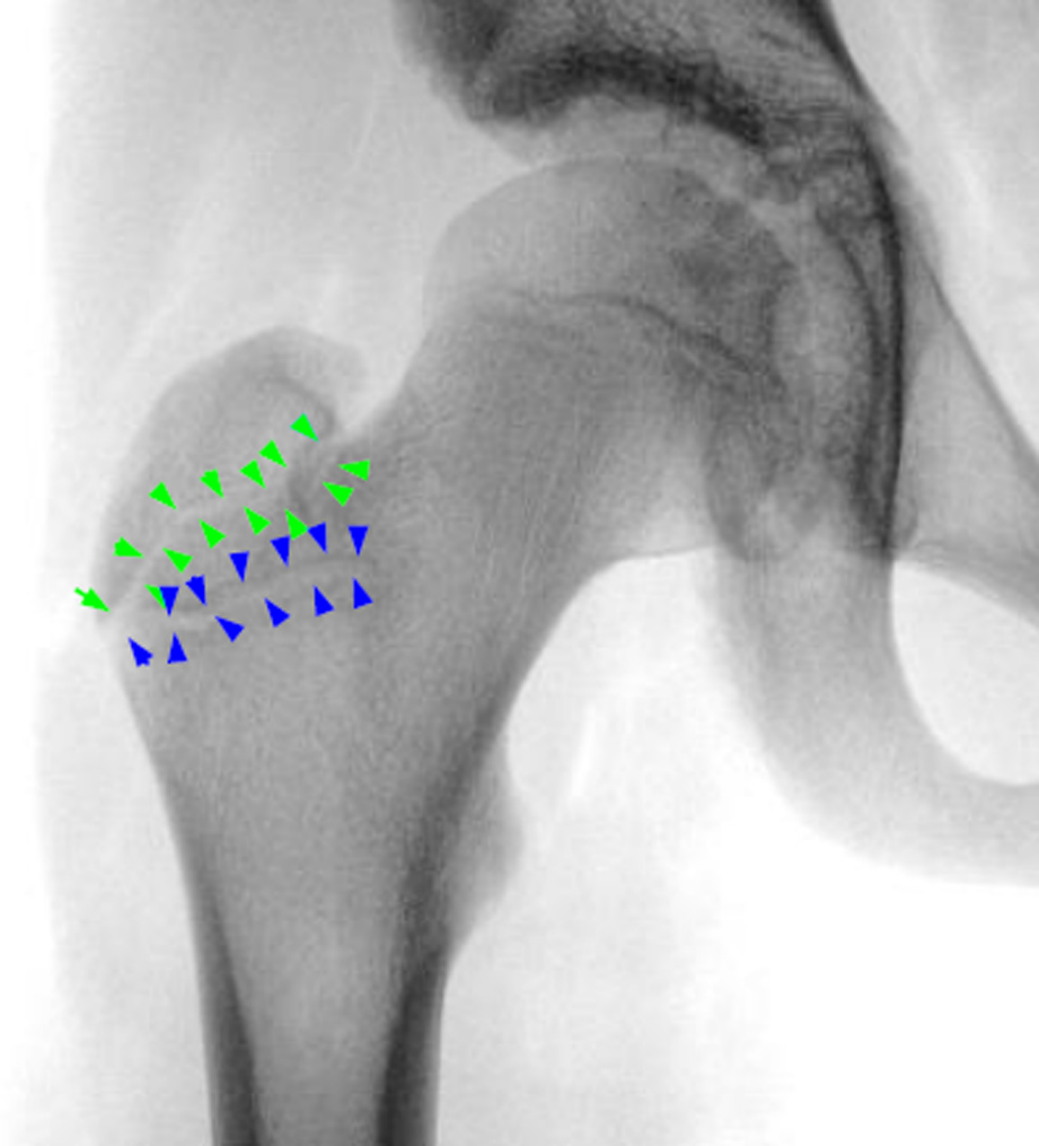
Conventional radiograph of a skeletally immature right proximal femur. The outlines of the ventral side of the greater trochanteric apophysis are marked with green arrows, the dorsal side with blue arrows.
